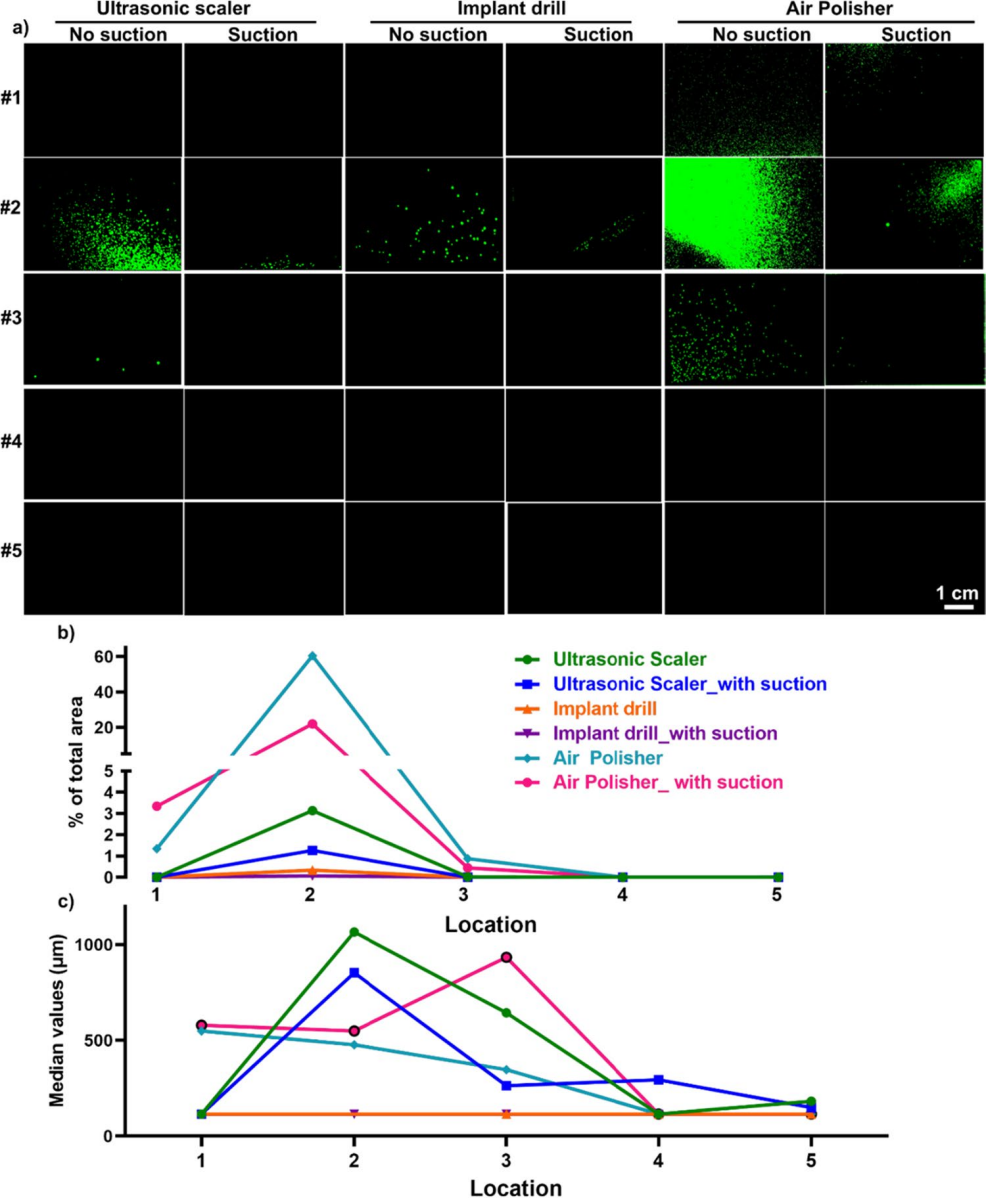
Representative images of particles on filter paper at various locations (a), the % of the total area (b), and the median particle size (c) for splatter particles. a HVS reduced splatter spread at 5 different locations: location #1 represents a right-handed dentist at 20 cm away from the source, location #2 represents a dental assistant at 15 cm away from the source, locations #3 and #4 represent the patient’s chest and body at 22 cm and 60 cm away from the source, and location #5 is at 120 cm away from the source. For locations, three dental AGPs generated the most splatter particles for a dental assistant (location 2). Among different AGPs, the air polisher generated the most splatter particles compared to the ultrasonic scaler and implant drill. b Quantification of splatters by dental AGPs by quantifying particles % of the total area for each filter paper. The application of HVS showed decreased splatter particles for all three dental AGPs. c The median size of splatter particles with and without HVS is larger than 200 μm
